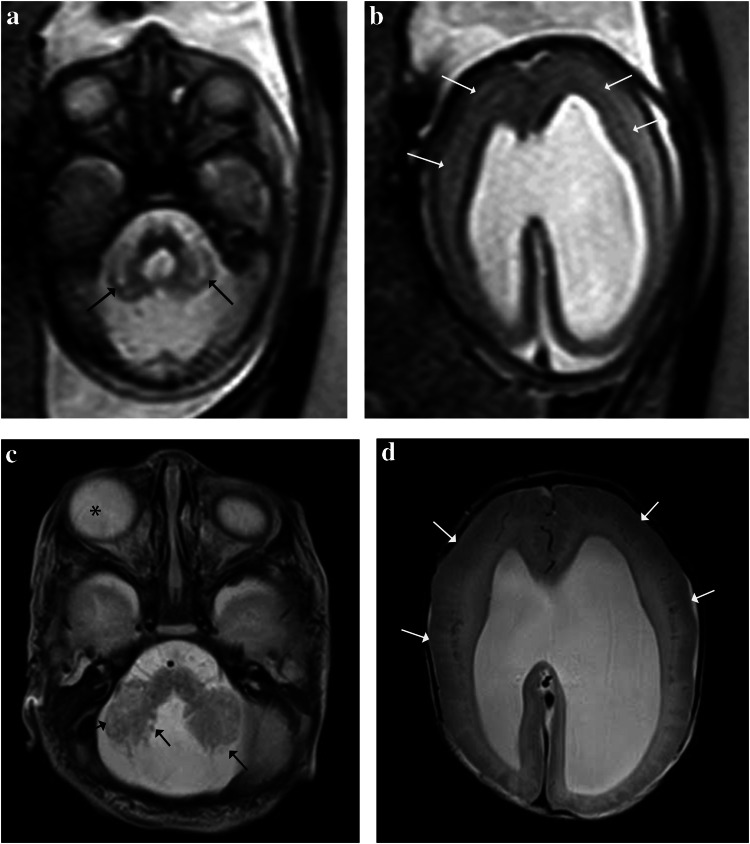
Cobblestone malformation in Walker-Warburg phenotype. Fetal MRI performed at 25 2/7 weeks shows features strongly suggestive of an alpha-dystroglycanopathy. Axial T2 SSFSE (a) through the posterior fossa shows small size of the cerebellum and subcortical hyperintense signal suggestive of cystic changes (*arrows*). Supratentorial axial SSFSE (b) demonstrates severe ventriculomegaly, simplified gyration for gestational age, and abnormal cerebral layering subcortical signal suggesting diffuse neuronal migrational abnormality. Postnatal brain MRI axial T2 through the posterior fossa at 1 week of age (c) shows severe dysmorphism and cystic changes of the cerebellar hemispheres (*arrows*). Buphthalmos (*asterisk*) is partially visualized on the right. Supratentorial axial T2 (d) shows ventriculomegaly and cobblestone malformation of the cerebral cortex (*arrows*)
